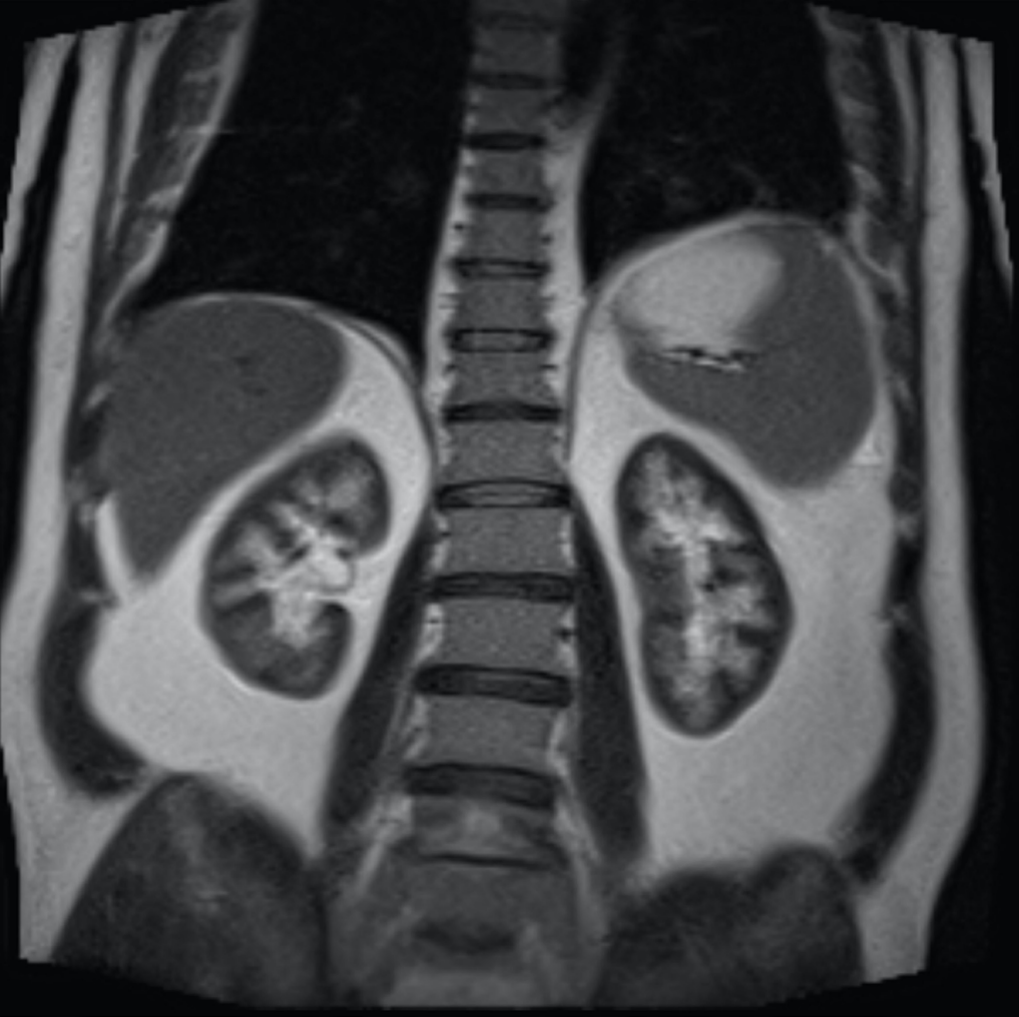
On MRI coronal T2-weighted images show prominent signal decrease of cortices of both kidneys and reversal of signal of cortex and medulla.
